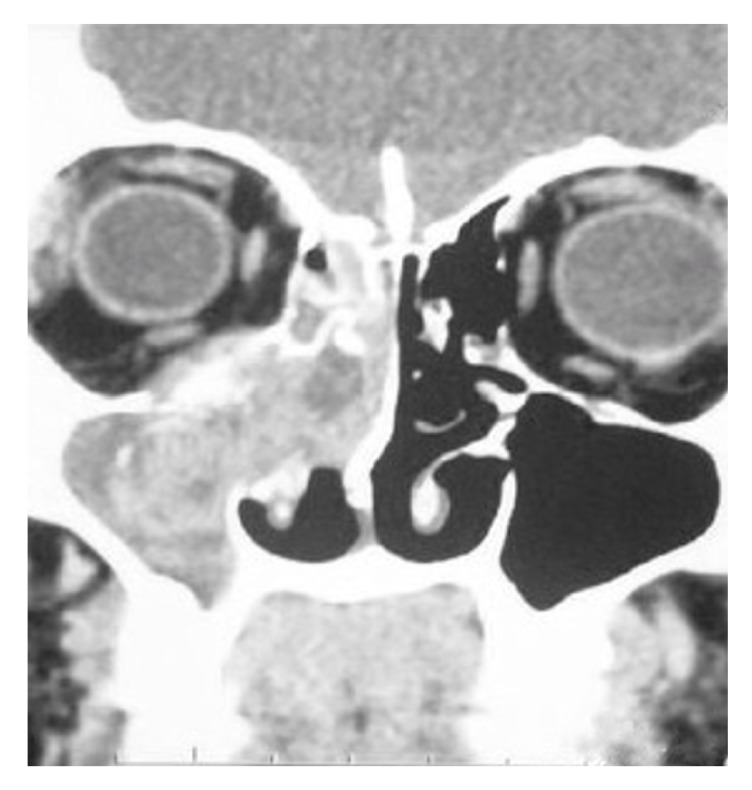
Computed tomography (CT) scan showing a mass causing an opacification of the right nasal cavity, ethmoid and maxillary sinuses.
